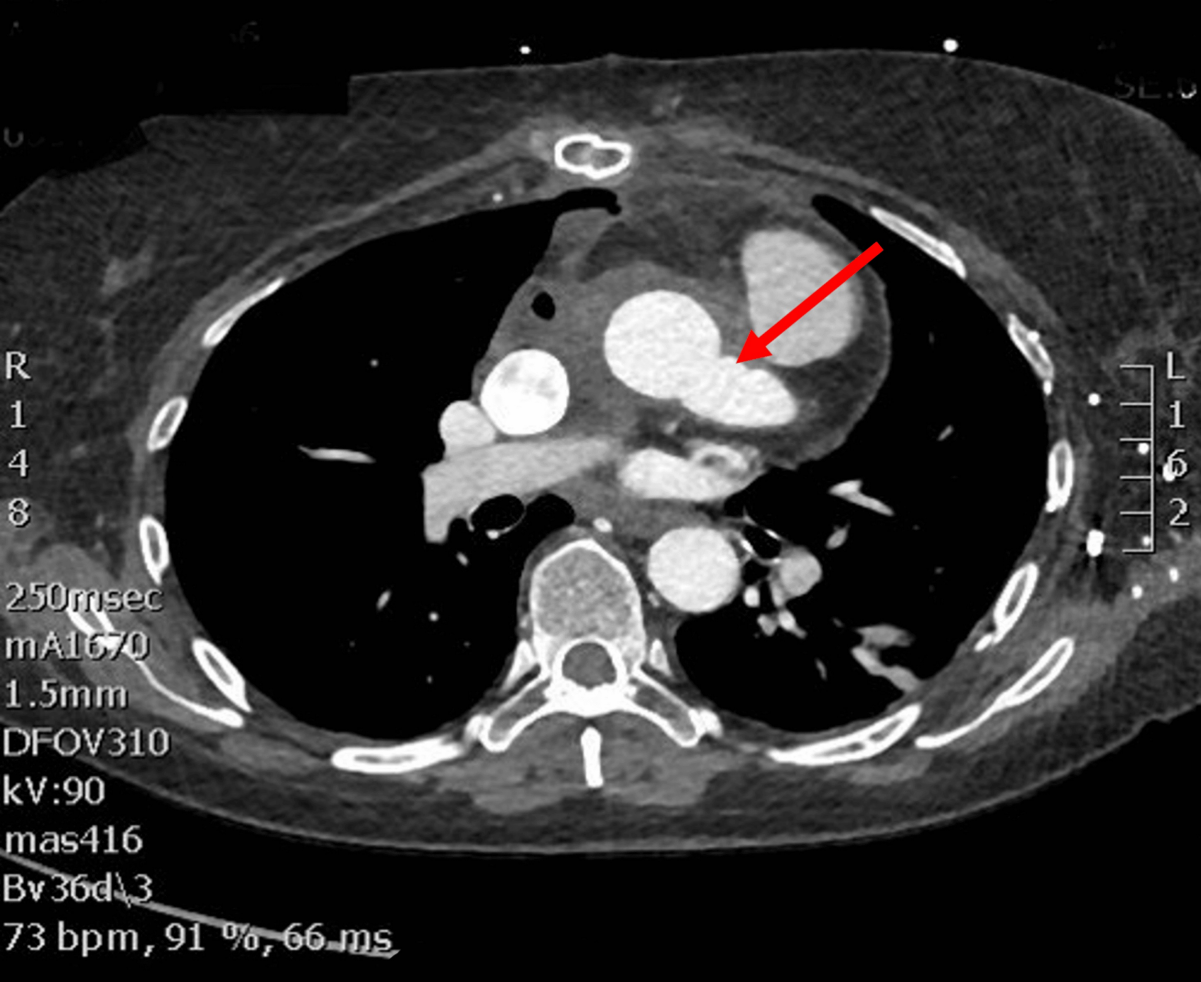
Postoperative computed tomography (CT) imaging demonstrating reconstructed left main coronary graft (red arrow).
